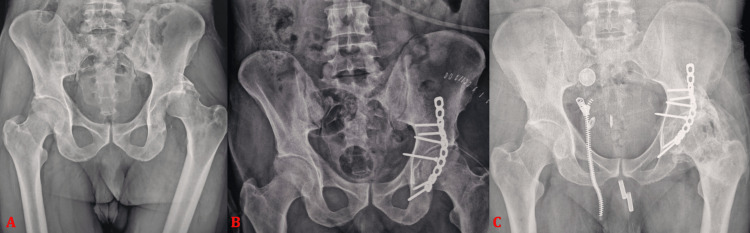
Anteroposterior pelvic radiographs showing (A) a posterior wall fracture of the left acetabulum associated with dislocation of the femoral head in a 38-year-old male patient. The patient underwent open reduction and internal fixation using a plate-and-screw construct, with postoperative control radiograph (B) demonstrating a congruent hip joint space. Follow-up at 23 months revealed progression to post-traumatic hip osteoarthritis with the development of heterotopic ossification (C).
